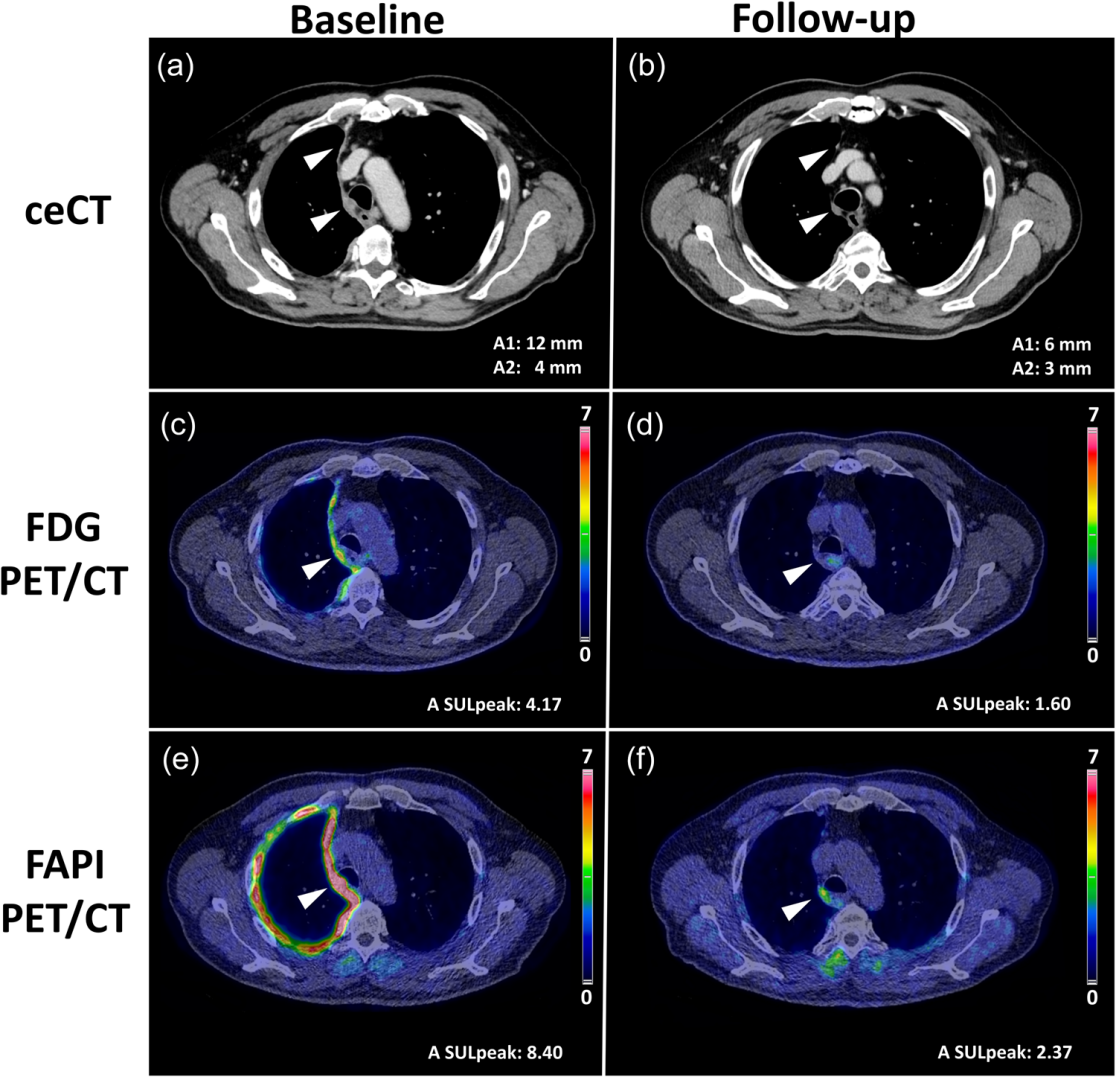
Imaging response assessment of Lesion A, located in the upper medial pleura, with ceCT (a, b), FDG PET/CT (c, d), and FAPI PET/CT (e, f).
